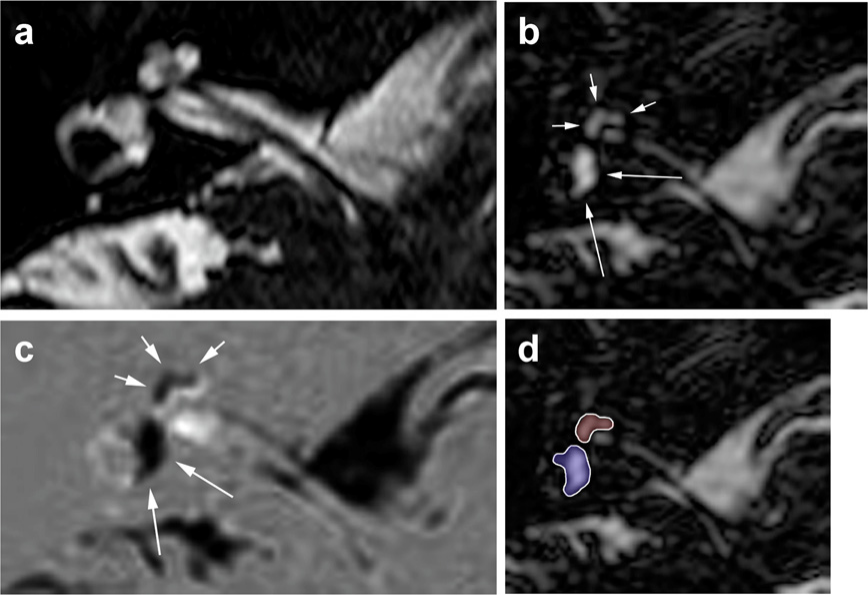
Images of a 57 year old woman with Menière’s disease, obtained 4h after i.v.-administration of a single-dose Gd-DO3A-butrol. (a) T2DRIVE-cisternography reveals the anatomy of the total lymphatic fluid of the right inner ear. (b) hT2w-FLAIR PEI with an inversion time of 2050 ms displays the endolymphatic fluid. (c) The HYDROPS (HYbriD of Reversed image Of Positive endolymph signal and native image of positive perilymph Signal) image, gained by subtraction of PEI from PPI, reveals the enlarged endolymphatic space as black area. Diagnosis after semi-quantitative analysis was a significant (II°) endolymphatic hydrops of the vestibule (long arrows) and the cochlea (short arrows). Volumetric assessment was performed by dedicated slice-by-slice-segmentation of the endolymphatic space in PEI (d) and the total labyrinth fluid space in T2DRIVE-cisternography. This patient showed an endolymph/total labyrinthine fluid-ratio of 50.51% (cochlea) and 54.3% (vestibule).
